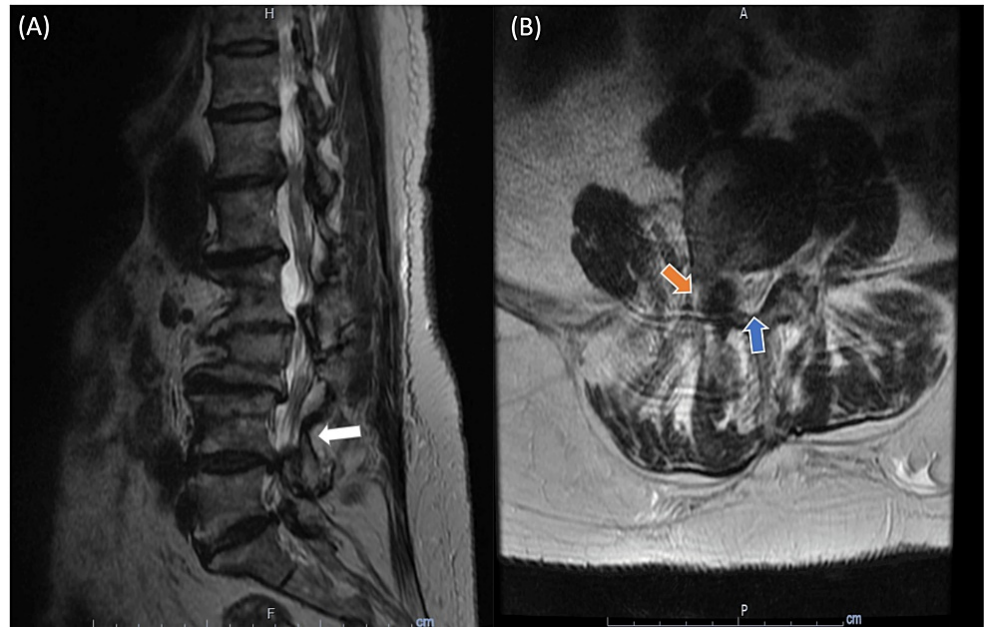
MRI of the lumbar spine (A) Sagittal T2-weighted MRI of the lumbar spine shows the L4-L5 level. (B) An axial T2-weighted MRI shows severe spinal stenosis and foraminal narrowing (orange arrow) with ligamentum flavum hypertrophy (blue arrow) at the L4-L5 level.
